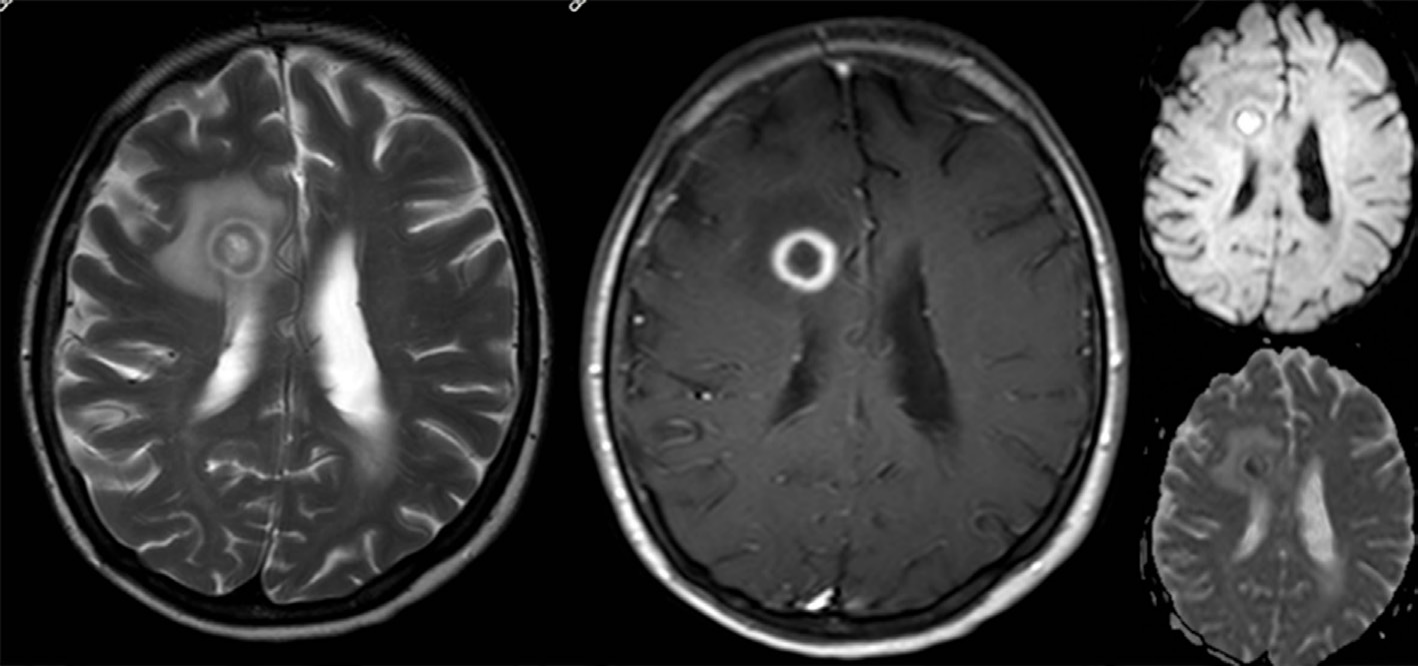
Brain magnetic resonance imaging in May 2014. There is an increase in size of the right frontal lesion with surrounding perilesional edema. T2-weighted image (left) demonstrates a hypo-intense rim with ring-enhancement after gadolinium (contrast-enhanced T1-weighted middle). At diffusion imaging (right panels) there is restricted diffusion in a part of the central area.
